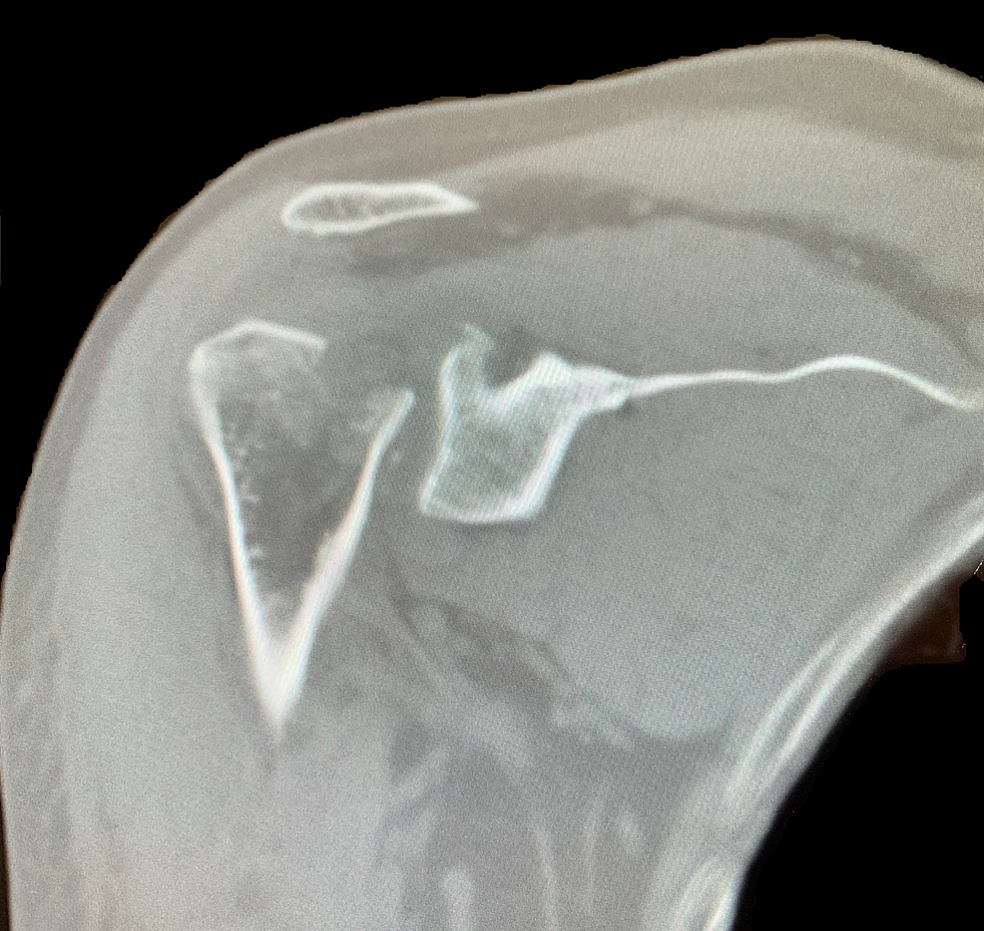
Coronal view of preoperative CT scan of the right shoulder demonstrating a four-part proximal humerus (PH) posterior fracture-dislocation.
